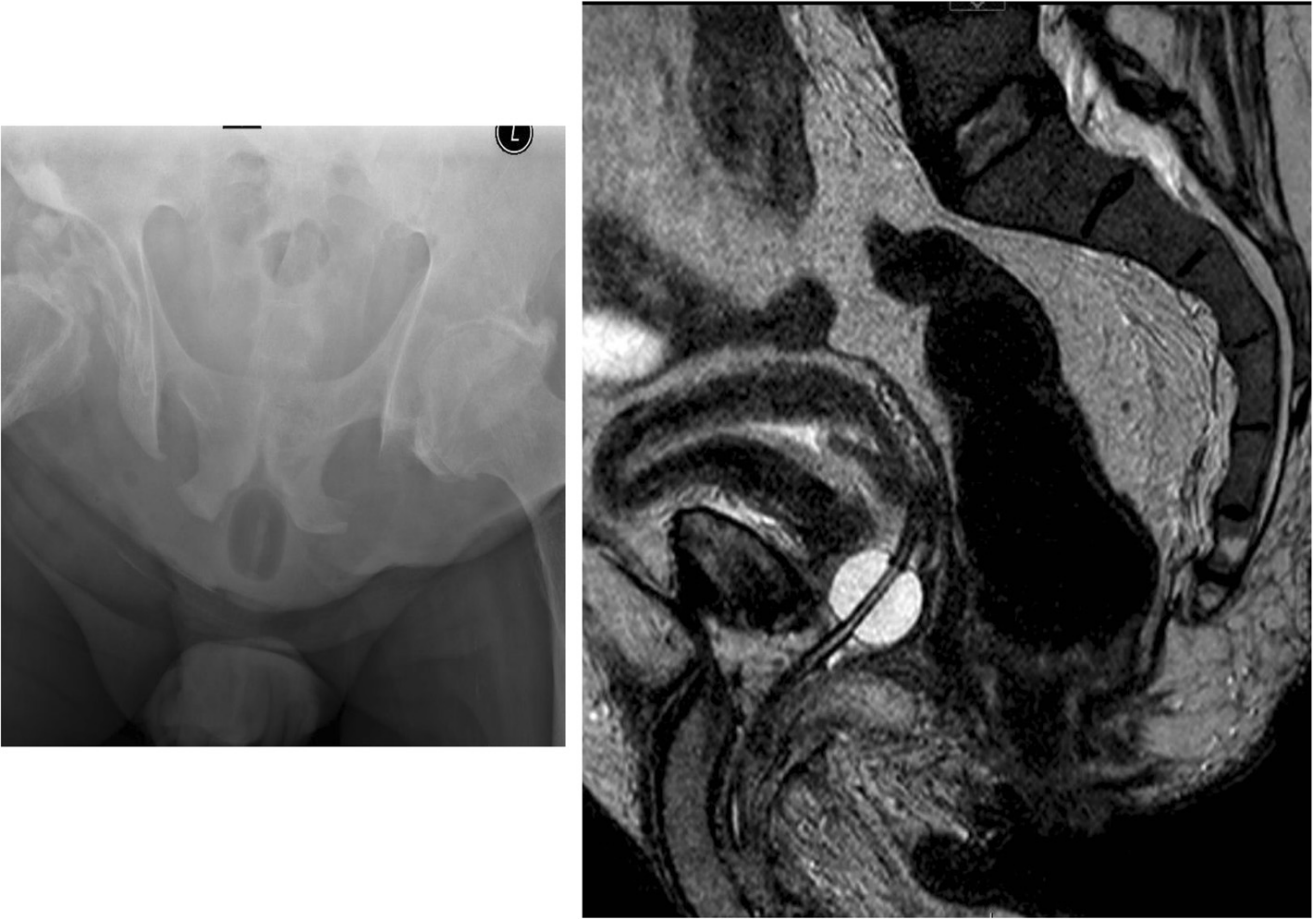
Case 3. Left panel: X-ray of pelvis taken after injecting air through the balloon channel of Foley catheter revealed that the balloon was located in urethra, well below ischiopubic ramus. Right panel: MRI of pelvis sagittal T-2 weighted image revealed balloon of Foley catheter in proximal urethra
